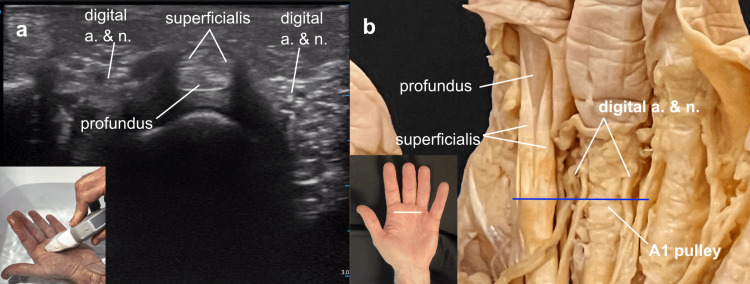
Stenosing tenosynovitis (trigger finger) anatomy Stenosing tenosynovitis (trigger finger) anatomy: (a) Short axis view of the region of the A1 pulley on ultrasound, and (b) in the cadaver demonstrating the relationship between the flexor tendon pulleys and adjacent neurovascular structures.
